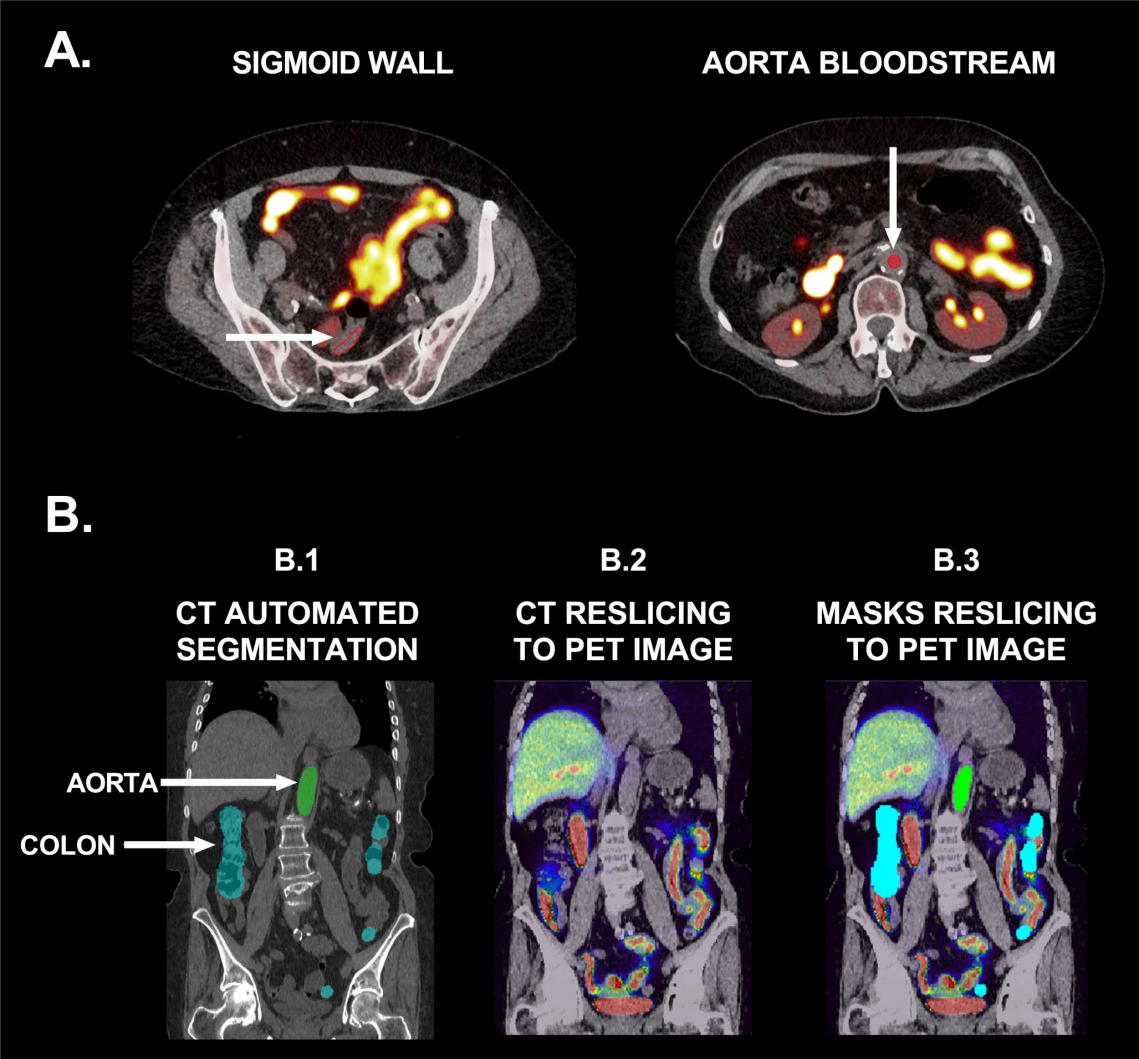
Manual and automated segmentation procedures of volumes of interest. Manual procedure (panel A): volumes of interest for the sigmoid wall and aorta bloodstream were manually drawn on CT. Automated procedure (panel B): (i) aorta (green) and colon (cyan) binary masks were automatically computed (panel B.1); (ii) CT images were resliced to PET space (panel B.2); (iii) the inverse transformation was applied to aorta and colon binary masks to match PET resolution, and the uptake values were computed (panel B.3)
